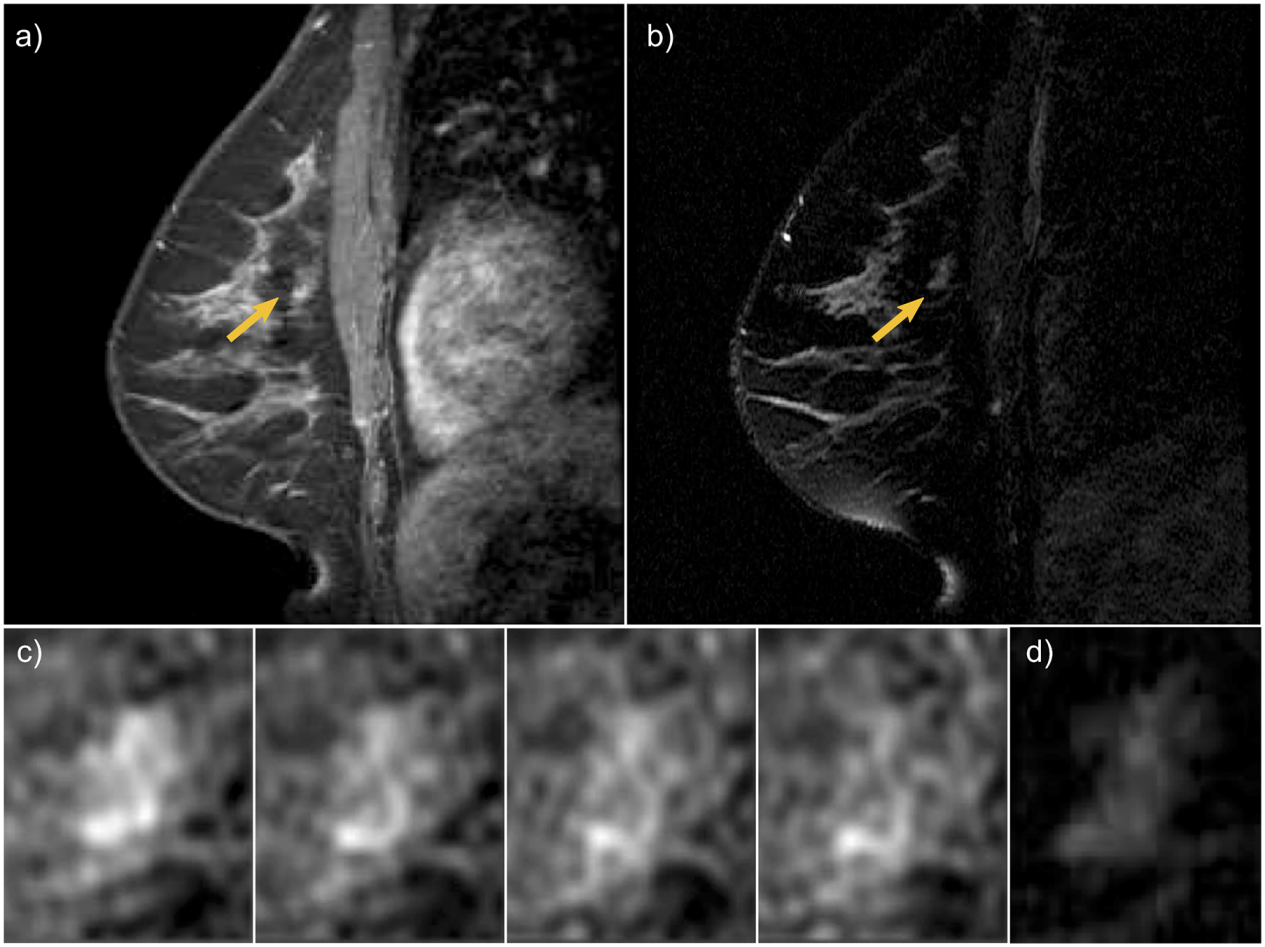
Invasive ductal carcinoma in a 36-year old high risk woman appeared as a focal non-mass, with persistent enhancement (BI-RADS score 4). a) T1w 4th post-contrast DCE-MRI. b) T2w MIR. c) zoomed progressive enhancement at each of the four post-contrast scans. d) zoomed lesion T2w appearance.
